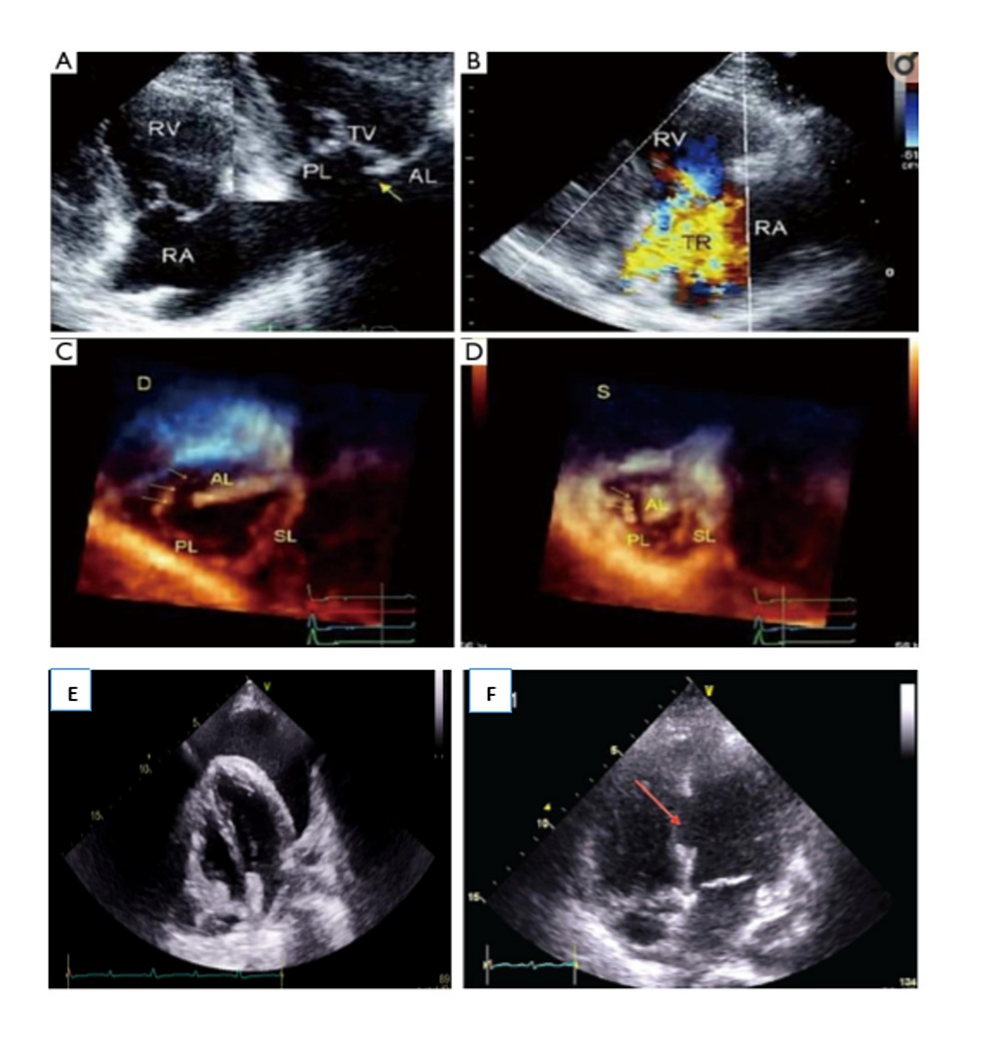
Transthoracic echocardiogram showing tricuspid valve injury (A, C, D), severe TR (B), pericardial effusion (E), and ventricular septal defect-red arrow (F).
